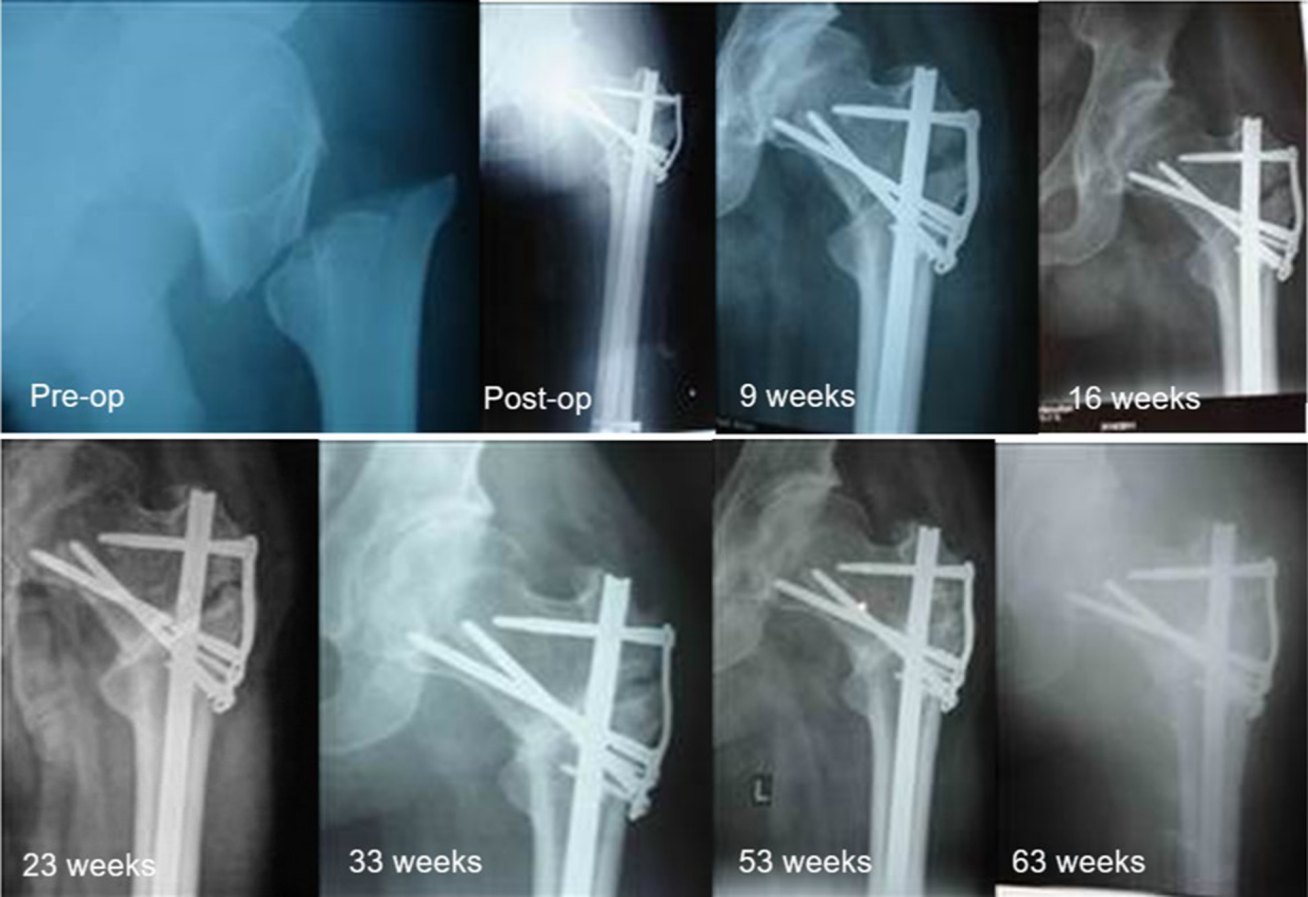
Radiographs of a 30 year old man with delayed union at 33 weeks after surgery but had painless full weight bearing at 9 weeks after surgery.
